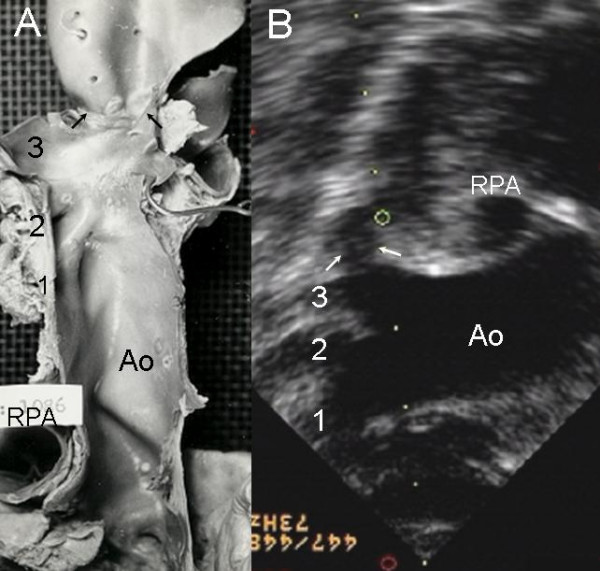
Coarctation of the aorta. (A) Aorta with narrow lumen (black arrows). (B) Suprasternal echocardiographic image reveals the site of the obstruction distal to the left subclavian artery (white arrows). RPA: Right pulmonary artery; 1-Brachiocephalic artery; 2-Left carotid artery; 3-Left subclavian artery.
